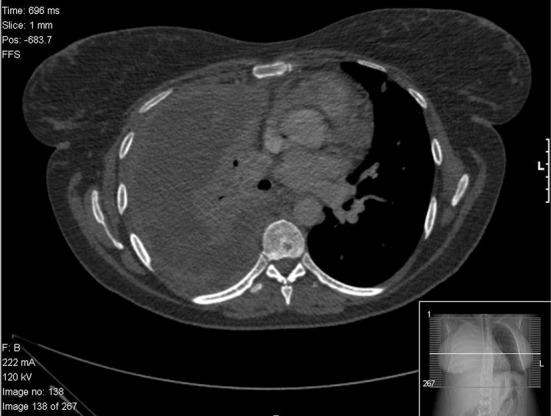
CT scan demonstrating pleural thickening, effusion, right lung collapse and left mediastinal shift.
